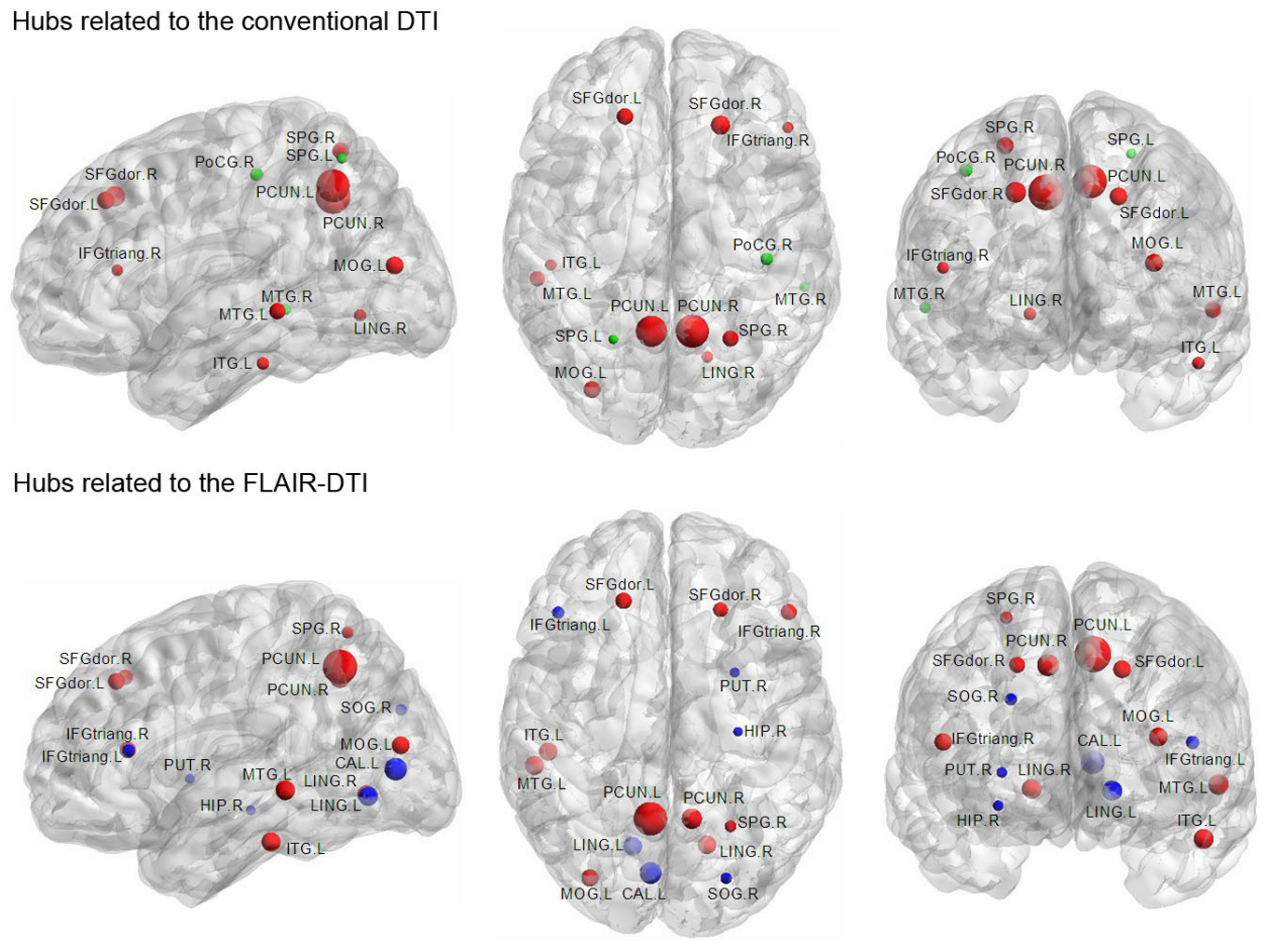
Rendering plot of the hub regions detected in the human brain anatomical networks for the conventional DTI (upper) and for the FLAIR-DTI (lower). Sixteen hub regions were identified in the anatomical network derived from the FLAIR-DTI datasets, whereas thirteen hub regions were derived in the network from the conventional DTI datasets. The size of the node represents the magnitude of the normalized betweenness centrality (see Table 3 for more details). Nodes in red represent hub regions shared by the networks derived from both types of DTI datasets. Nodes in green (blue) represent the hub regions specific to the network derived from the conventional DTI (FLAIR-DTI) datasets.
